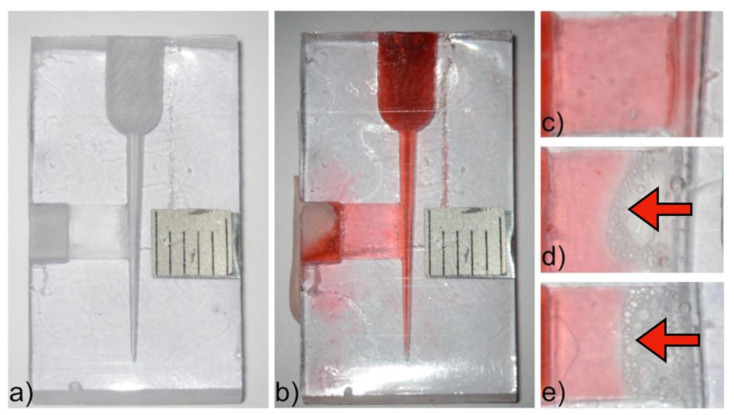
(a) A finger spreader and a matrix band were used to create models representing a root canal with a lateral isthmus. (b) The model was filled with gelatin mixed with red food dye. (c) Irrigation with physiological saline was used as a negative control. (d) When NaOCl was used as an irrigant and activated with am ultrasonically oscillating tip for 30 s, 43.2 ± 8.7% of isthmus surface was cleaned. (e) Adding HEDP to NaOCl did not affect cleaning efficacy (47.9 ± 9.4% cleaned, *p* > 0.05), as can be appreciated by comparing panels (d) and (e) (arrows).
